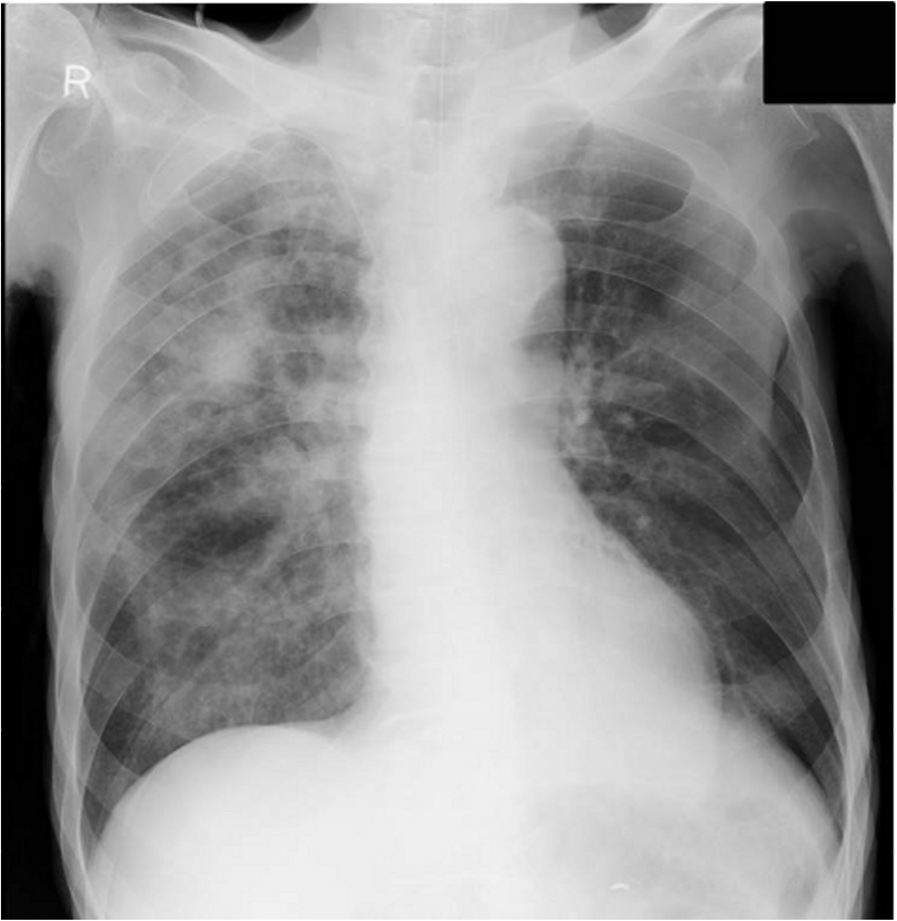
Multiple nodules and ground-glass opacities. The chest x-ray showed multiple nodules in the right upper lung field, and mixed ground-glass and airspace opacities in the entire right lung.
